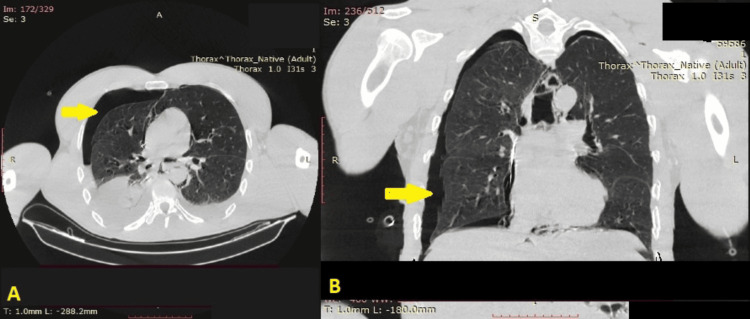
A) Axial and B) coronal CT views of bilateral hydropneumothorax and mediastinal emphysema (arrows) CT: computed tomography
